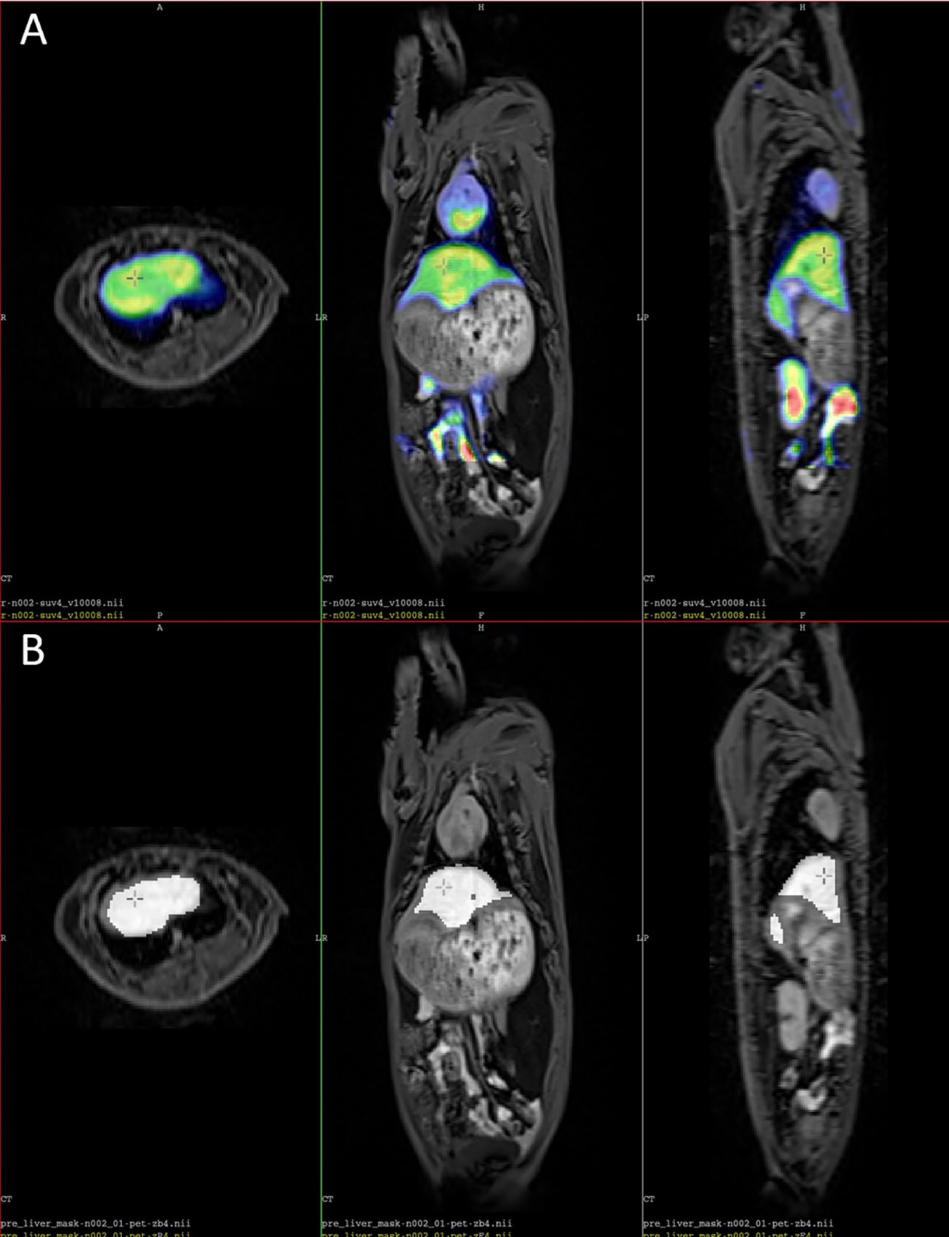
(A) Image fusion of ^18^F-FDG PET and T1 weighted MR images in three orthogonal sections. (B) The mean liver volume (MLV) generated with the region growing technique.
